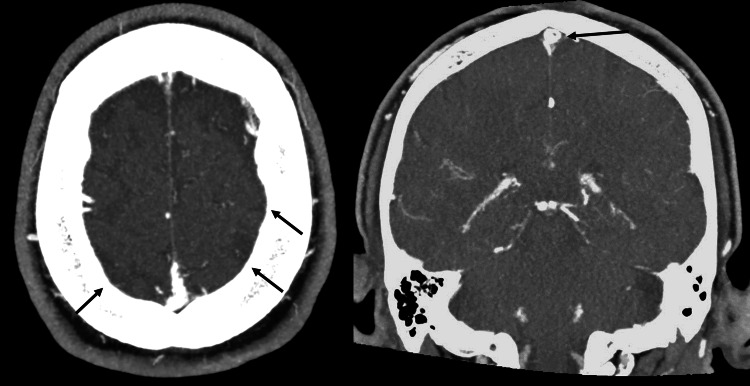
A CT venogram image showed bilateral cerebral venous sinus thrombosis. Note the image on the left is similar in appearance to the computed tomography angiogram (CTA) performed on 15/9/24 in Figure 1. A subtle filling defect was visible in the right lateral superior sagittal sinus, suggestive of a non-occlusive thrombus indicated by the arrow on the right panel.
